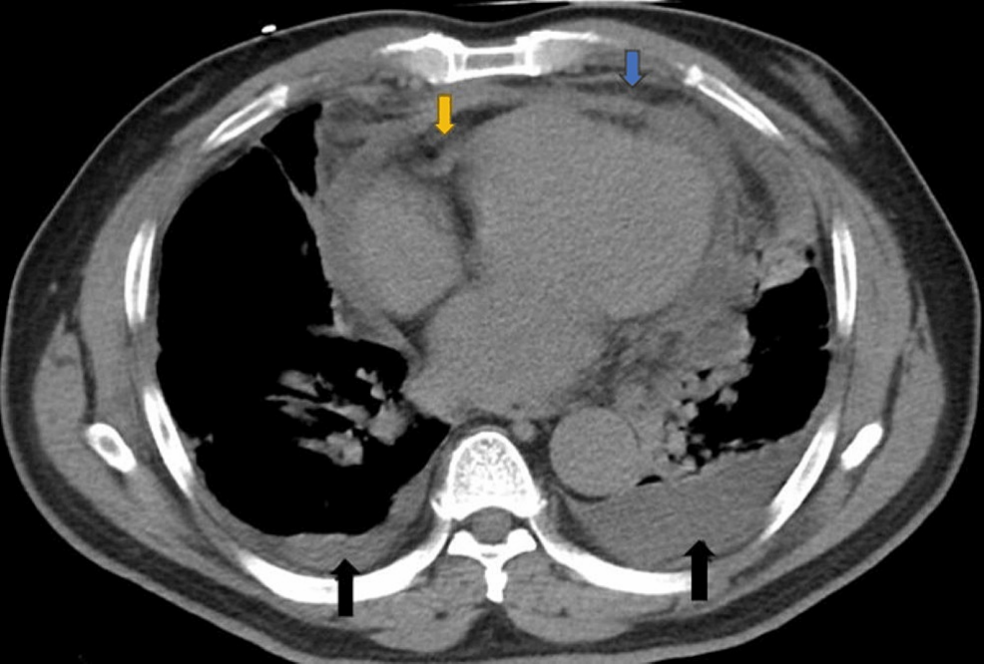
CT chest showing bilateral loculated pleural effusion, with left greater than right (black arrows), pericardial effusion (yellow arrow) and mediastinal fluid (blue arrows).
